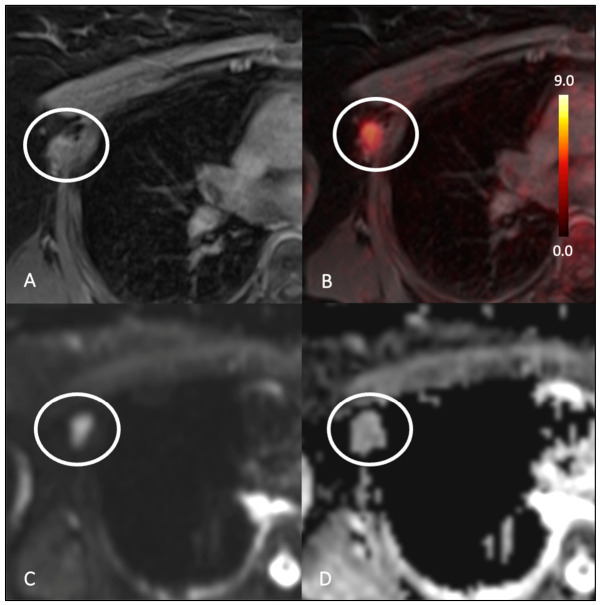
Right axillary lymph node metastasis in a breast cancer patient (tumor stage according to TNM classification: cT2 N1 M0). Gadolinium-enhanced T1 image (A) and fusion image of [^18^F]FDG-PET/MRI (B) showing strong, focal tracer signal (FDG+). Focal area of high signal intensity on b1000 DWI image (C) and with low ADC value (0.80 × 10^−3^ mm^2^/s) on the ADC map (D).
